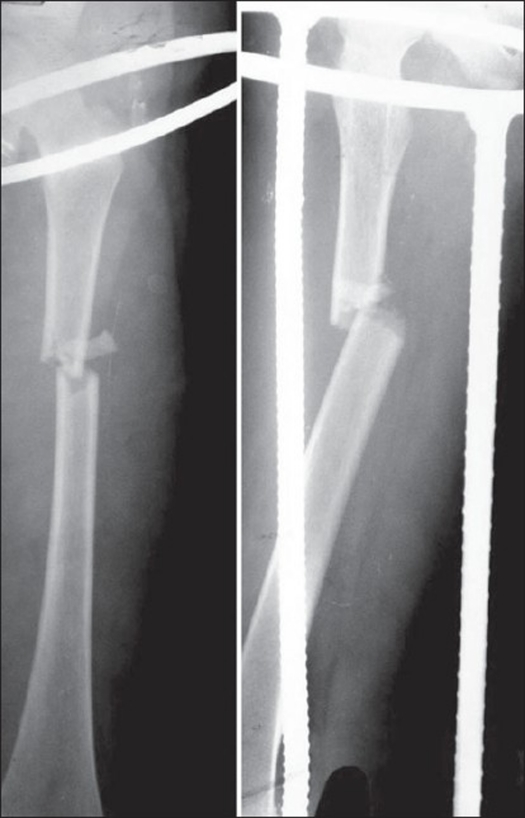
Preoperative x-ray of femur (AP and Lat View)
